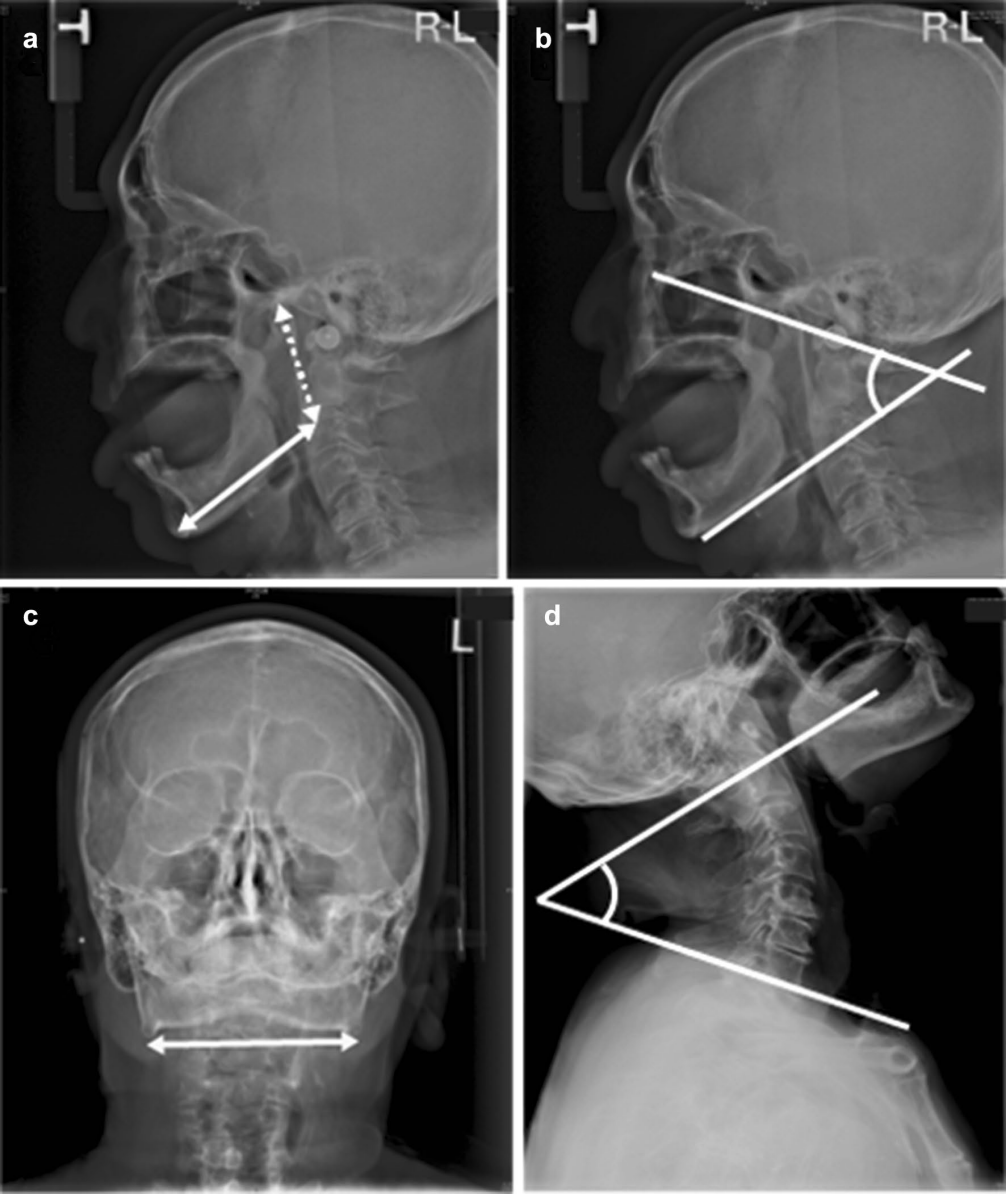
The cephalometry and cervical spine radiograpy. Mandibular bone parameters were measured by the cephalometry, such as mental–gonion distance (a; solid line), articulare–gonion distance (a; dot line), aperture angle (b), and intergonion distance (c; solid line). Cervical lordosis degree was measured by cervical spine radiography (d)
